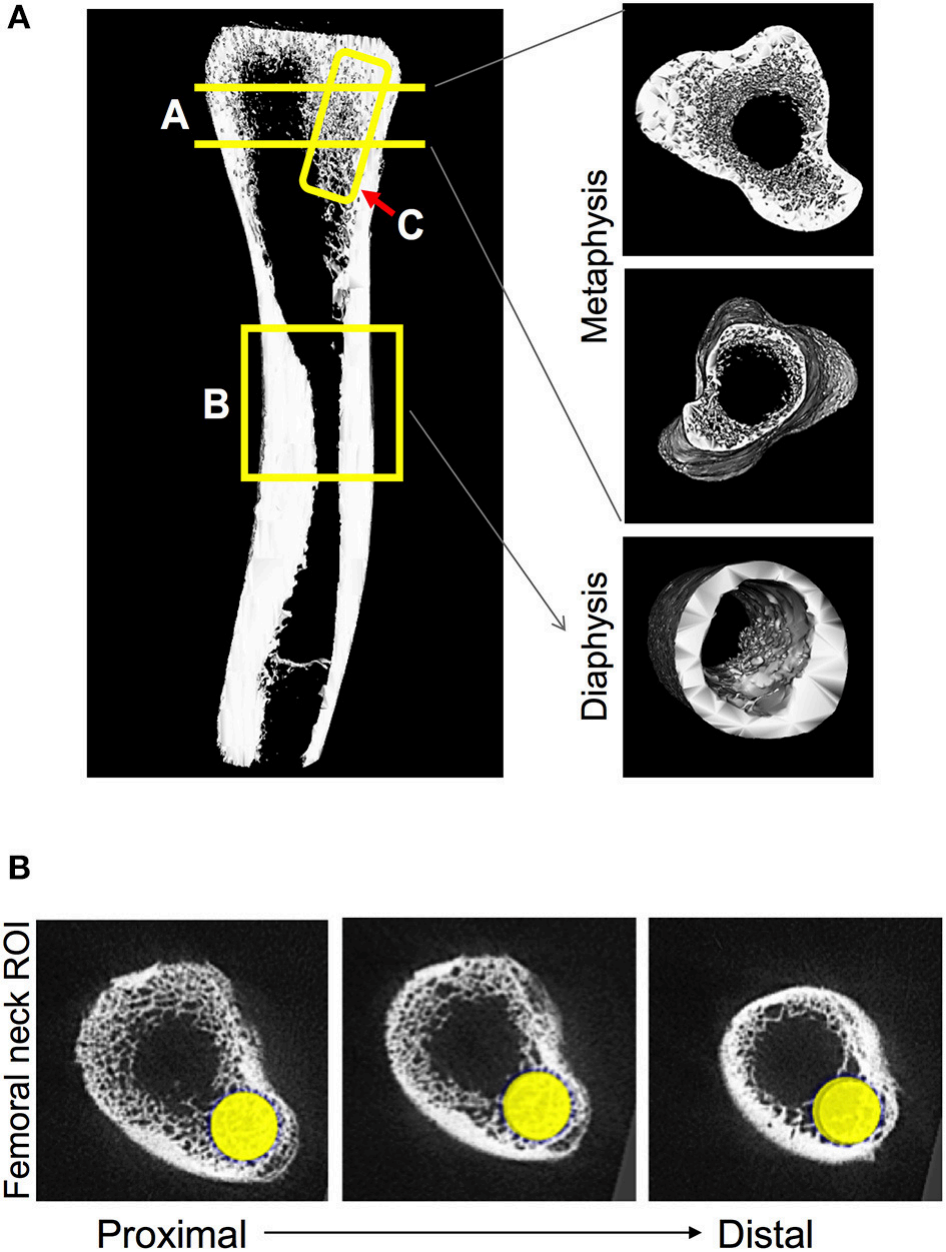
Regions of chicken femoral bone analyzed by microcomputed tomography. (A) Isosurface image indicating regions of bone examined: A-Region = trabecular bone in the metaphysis that is measured at 10% of the total femur length starting from the growth plate and moving distally. B-Region = diaphyseal cortical bone that is measured at 50% of the total length of the femur by a 7 mm cube region of interest. C-Region = femur neck trabecular bone region of interest (ROI) measured by utilizing a 2 mm diameter × 4 mm length cylindrical tube. (B) Three sections, proximal-to-distal, indicating the location of the cylinder used for the femur neck ROI.
